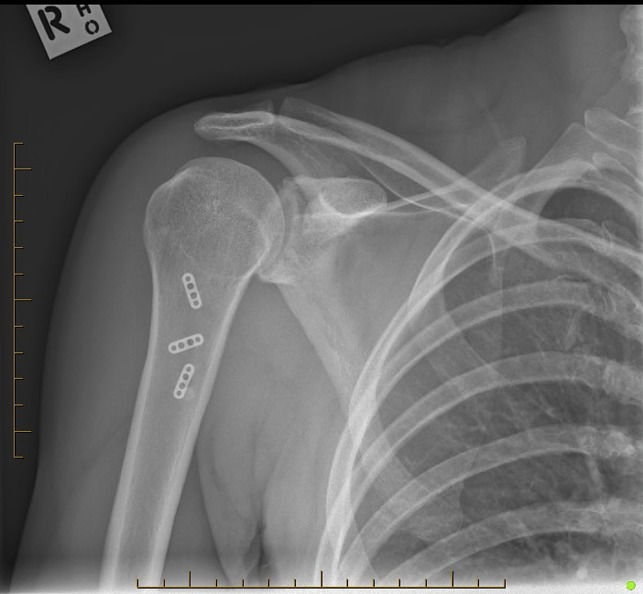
Postoperative AP radiograph.
